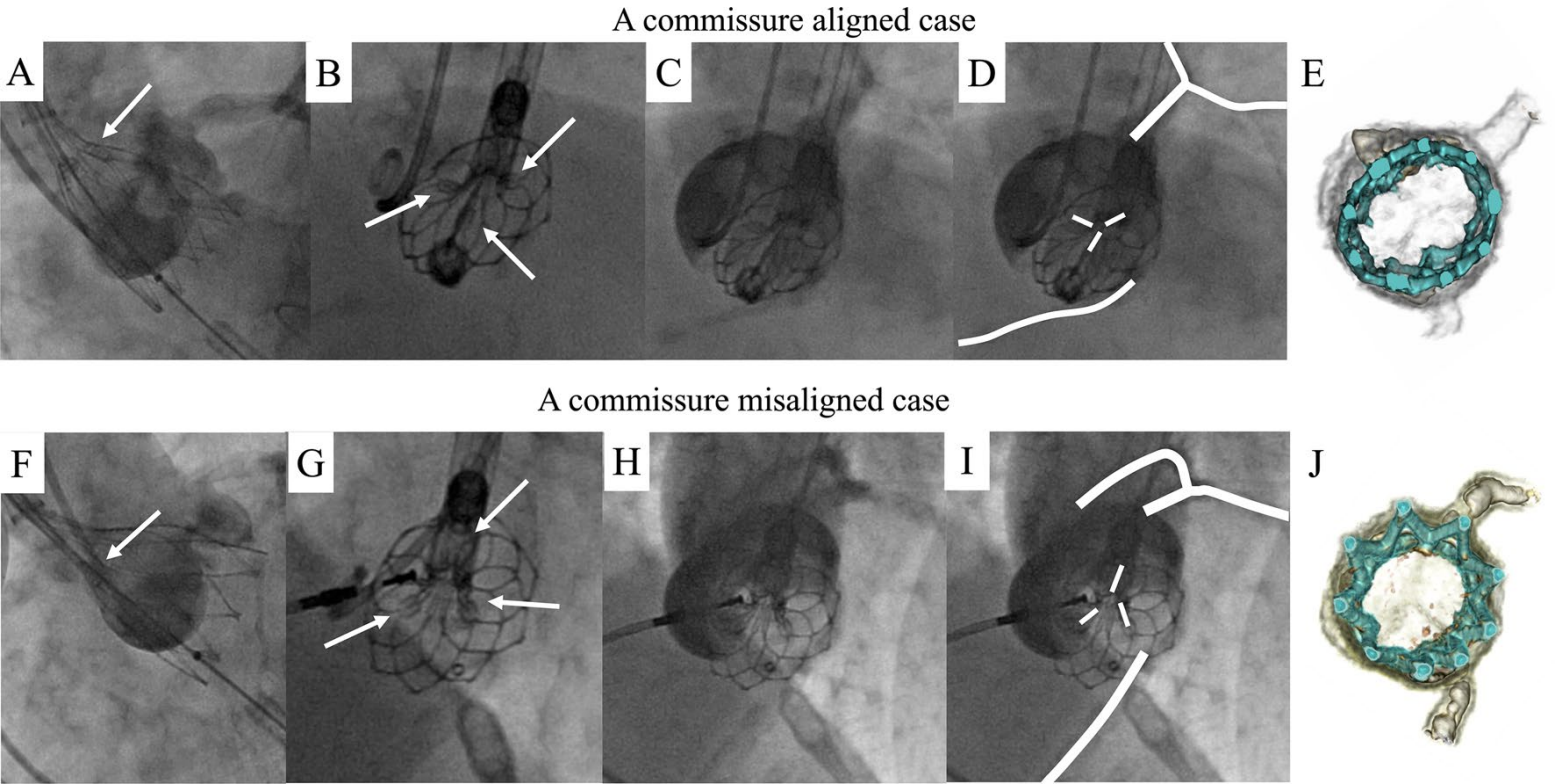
Using the en-face view, implant the Navitor valve. The upper and lower portions represent the aligned and misaligned cases, respectively. A Aortography with the Navitor heart valve at 80% development and recognizable CAFs (arrow). B–D Angiograms taken from the en-face view. White line traces both coronary and CAFs. E Commissure alignment was confirmed with the postoperative CT volume rendering (VR) images. F Aortography with the perpendicular view in the misalignment case. G–I CAF overlapped the coronary ostium using the en-face view, resulting in misalignment. J Postoperative CT confirmed misalignment. *CT* computed tomography; *CAF* commissure attachment features
